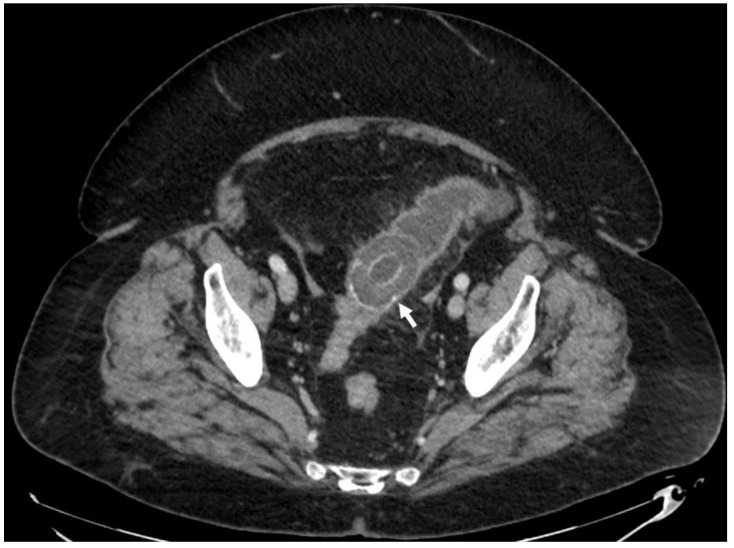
69-year-old female. Abdominal CT scan, axial plane, portovenous phase. The gallstone that migrated through cholecystocolic fistula is obstructing the lumen of sigma (arrow).
